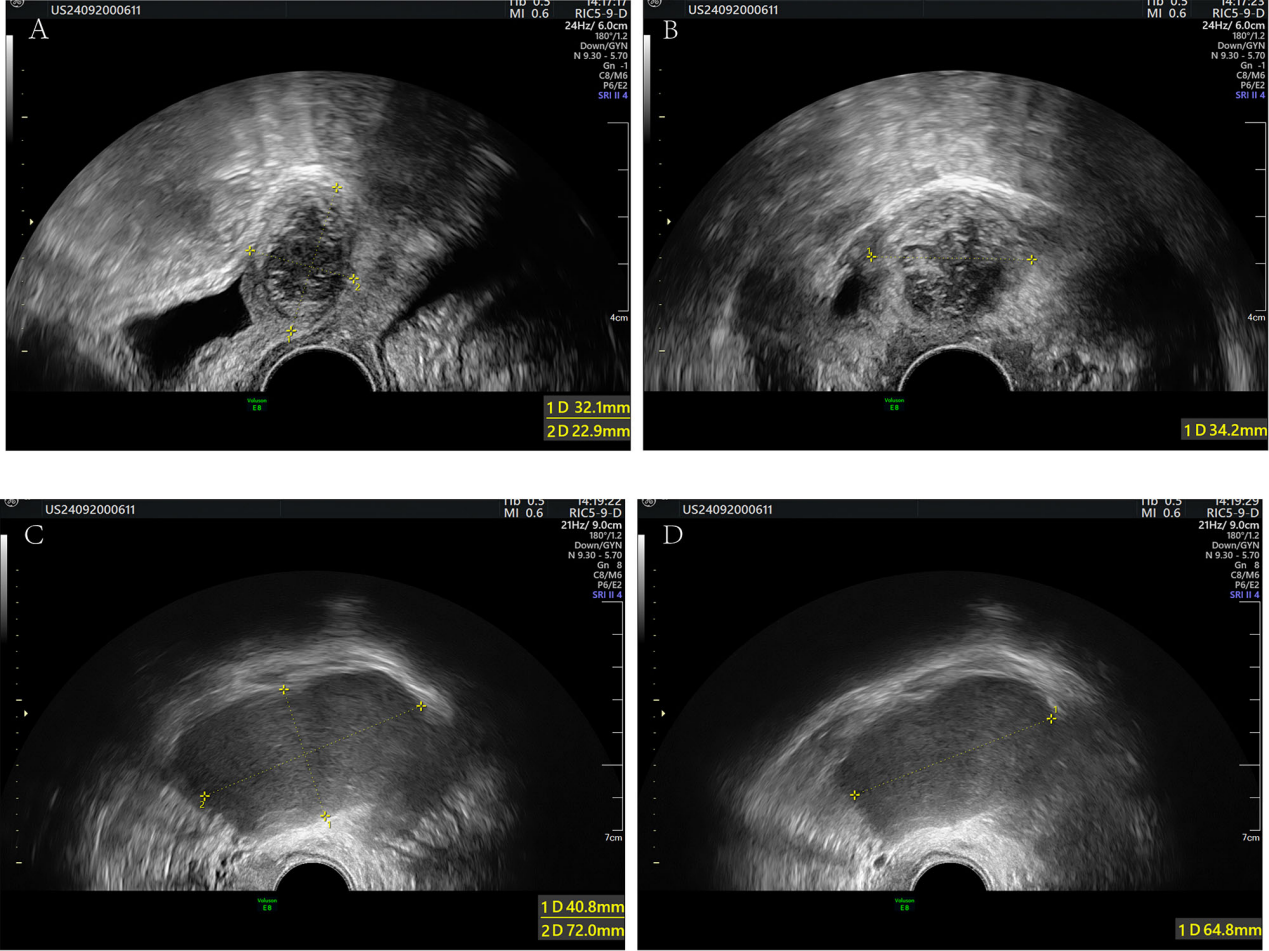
Transvaginal ultrasound showed a hypoechoic structure between the cervix and bladder, measuring 32X34X23mm (A, B). and the right ovary showed hypoechoic areas measuring 42X72X64 mm (C, D).
